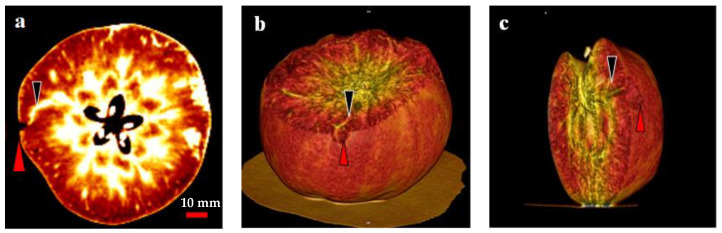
Computed tomography scanned images of a cork spot-like physiological disorder (CSPD) affected non-bagged ‘Kurenainoyume’ apple fruit. (a) 2-dimensional image; (b,c) 3-dimensional images. Red and black arrows indicate CSPD lesions and vascular bundles, respectively.
